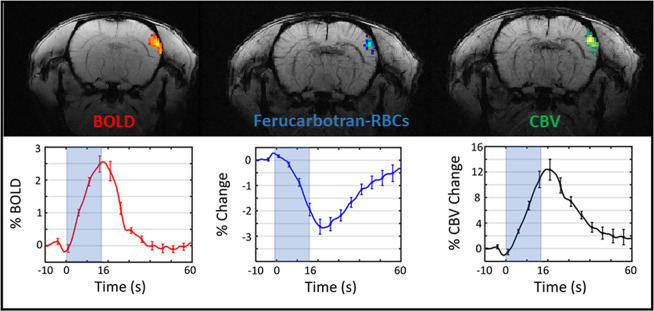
Representative pre and post functional MRI data (∼16.4
